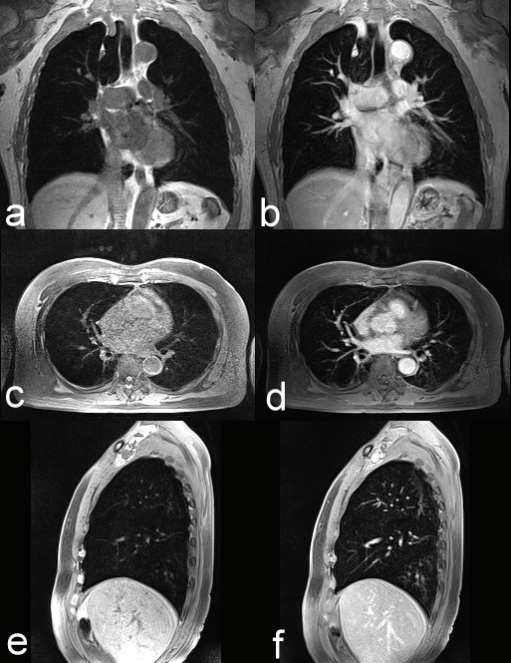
Comparison of VIBE images from MRI without and with the use of contrast. The (a) noncontrasted coronal, (b) contrasted coronal, (c) noncontrasted axial, (d) contrasted axial, (e) noncontrasted sagittal, and (f) contrasted sagittal VIBE images can display clear branches of pulmonary vessels and bronchial tree with minimal pulsation artifacts in normal lung parenchyma.
